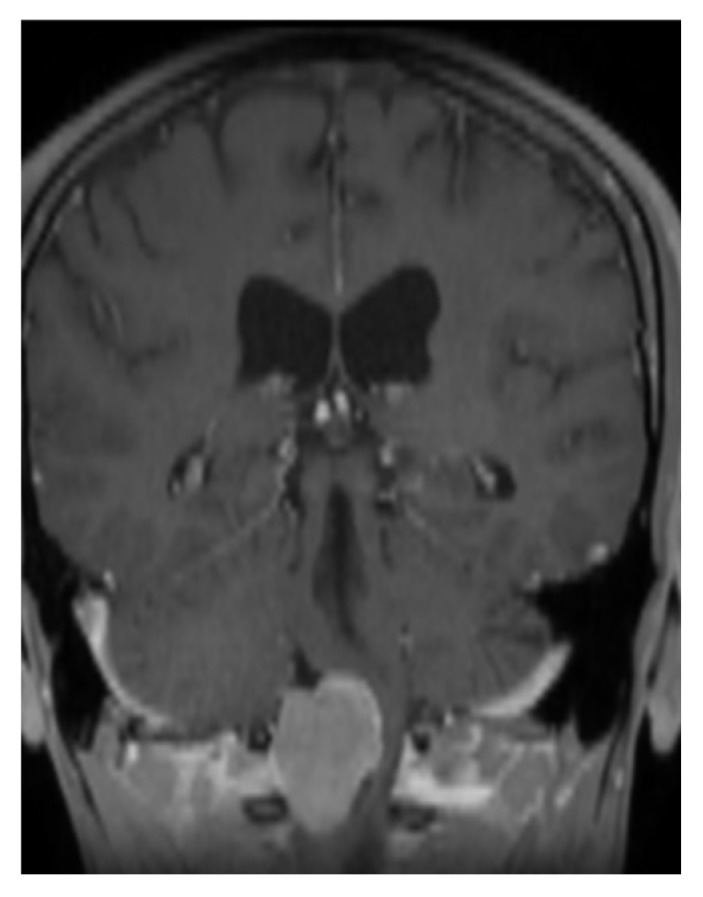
Single coronal scan of a right-sided foramen magnum meningioma with mild brainstem modeling. MRI T1 + C.
